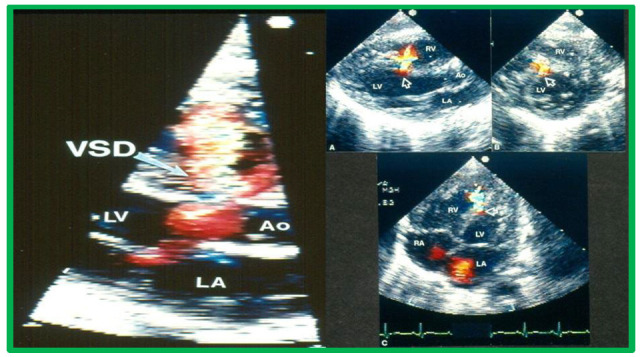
Echocardiographic imaging of perimembranous VSDs.
